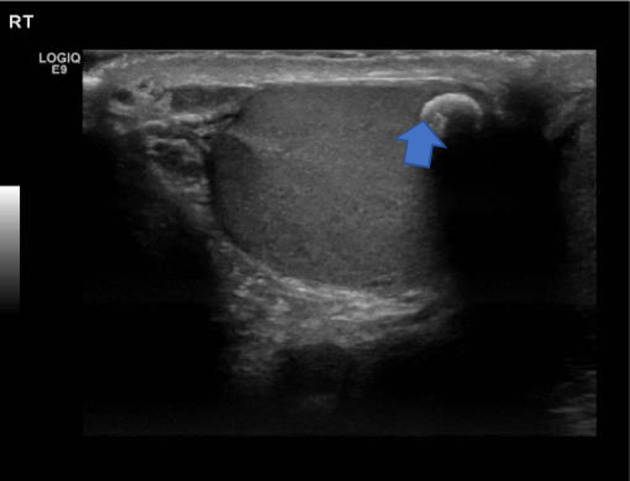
Right testicular ultrasound showing a large calcification at the inferior aspect of the testis (arrow).
